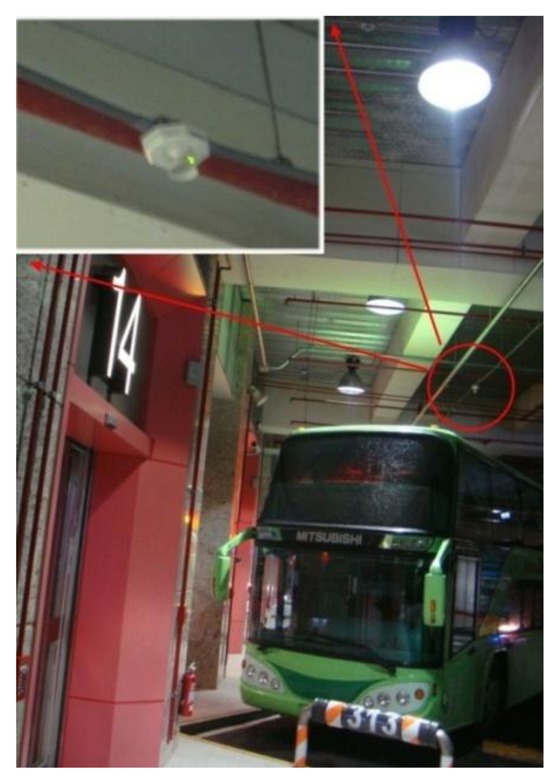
The actual ultrasound transmitter is located approximately 1m from the buses.
